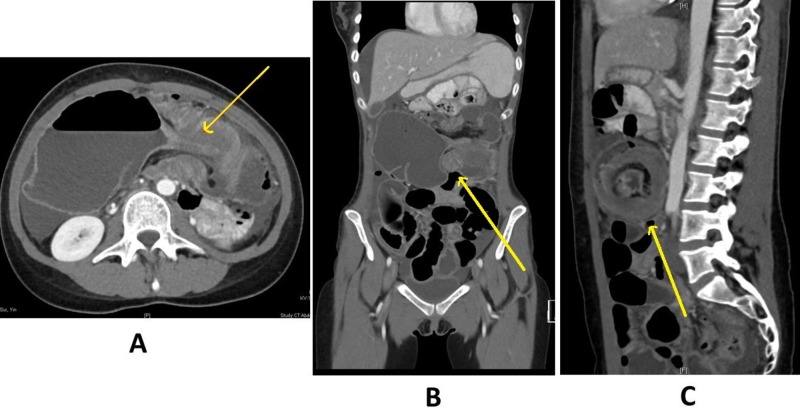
CT scan of pathological couplet Contrast-enhanced abdominal computed tomography (CT) scan showing the combined colocolic intussusception and cecal bascule in the (A) axial, (B) coronal, and (C) sagittal planes. The yellow arrow shows the lead point in the different planes.
